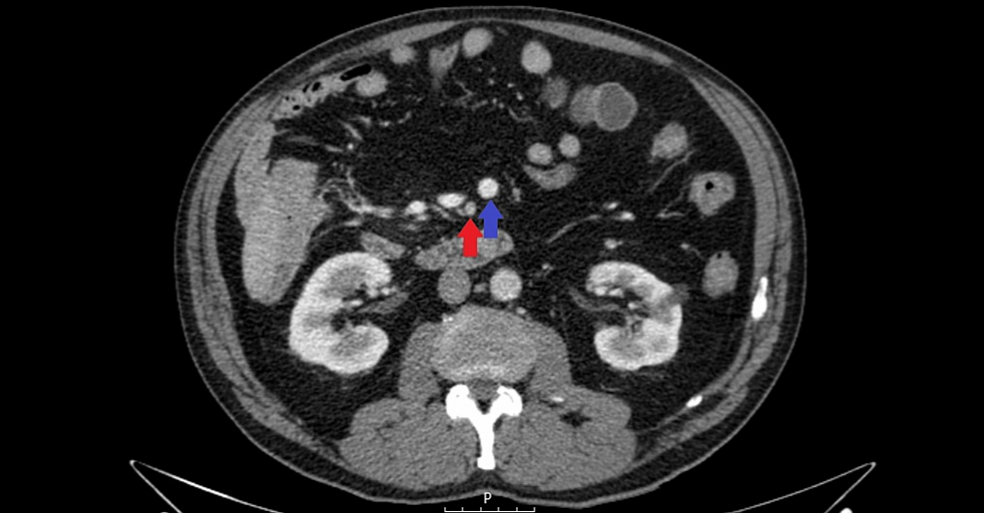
Axial contrast-enhanced CT scan showing an abnormal relationship between superior mesenteric vessels, with the SMA (red arrow) positioned to the right of the SMV (blue arrow). SMA: superior mesenteric artery, SMV: superior mesenteric vein
